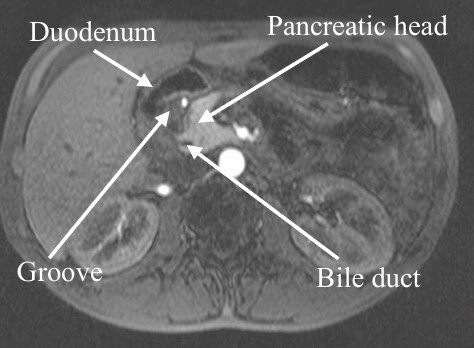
T2-weighted MRI of a patient with groove pancreatitis showing thickening of the groove, a pancreas with normal appearance, and a non-dilated common bile duct (arrows).
